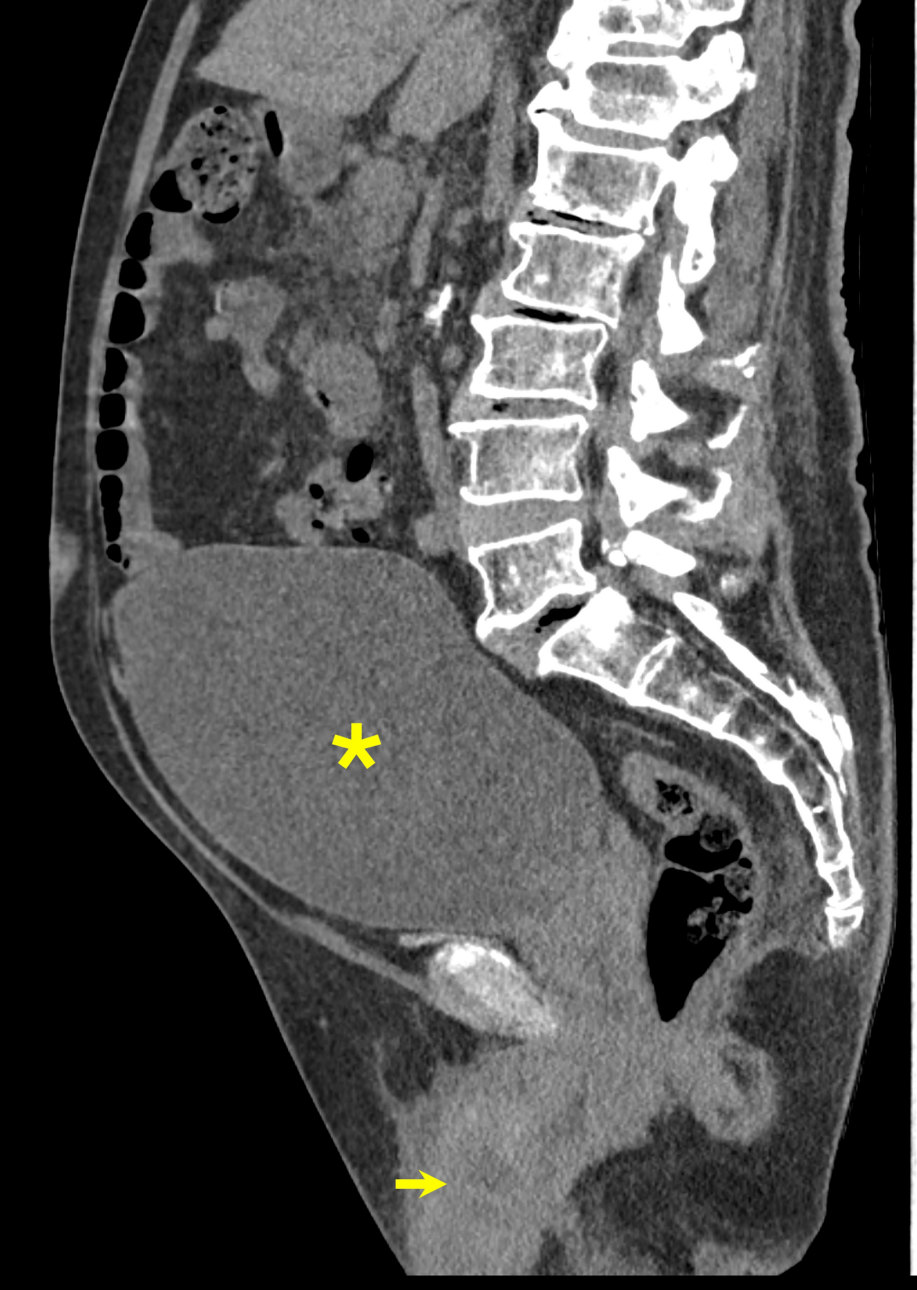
Sagittal non-contrast CT scan of the abdomen and pelvis The image demonstrates a severely distended urinary bladder (asterisk) and multiple irregular penile masses (arrow) CT: computed tomography
